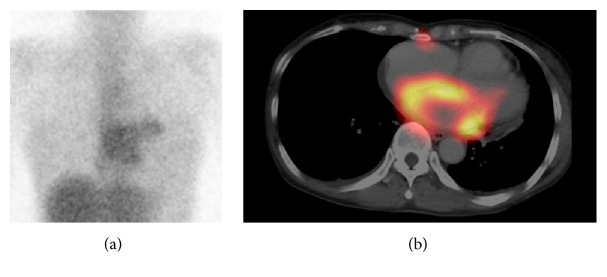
Gallium-67 imaging was performed 72 hours after intravenous injection with 74 MBq of gallium-67 citrate. (a) Frontal planar image of gallium-67 scan showed abnormal accumulation of radioisotope in the heart. (b) Single photon emission computed tomography/computed tomography fusion image of gallium-67 scan showed diffuse abnormal accumulation of radioisotope in the thickened left atrial wall.
